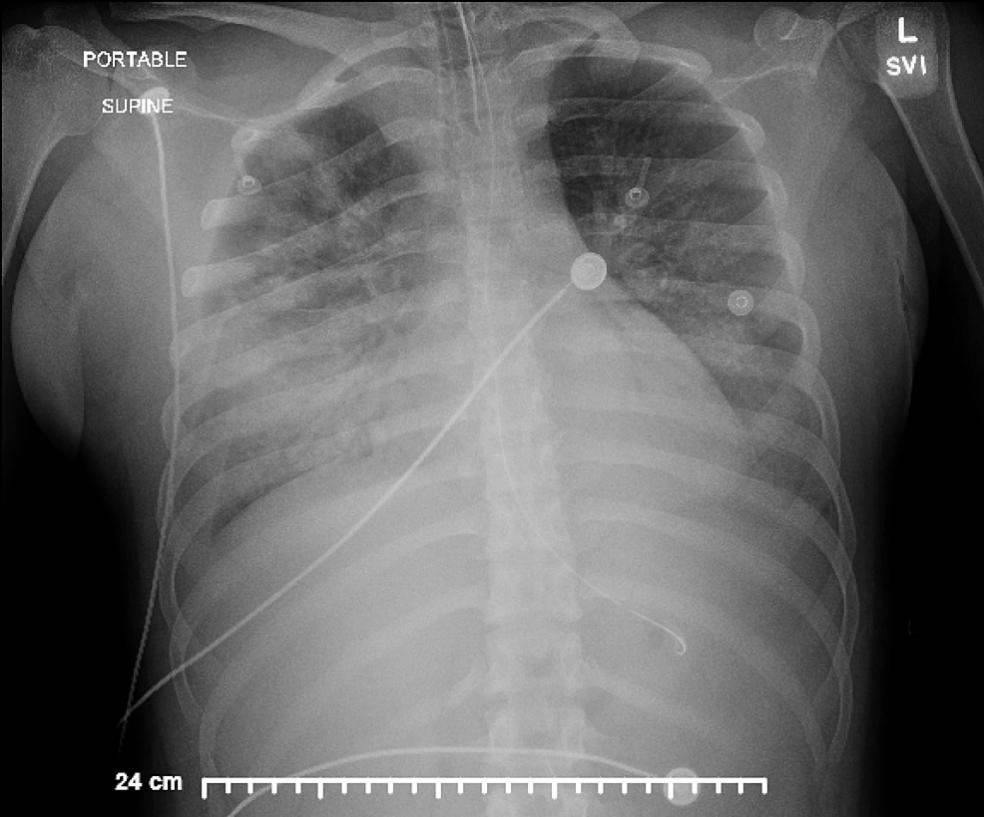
The chest X-ray showed bilateral lung opacities.
